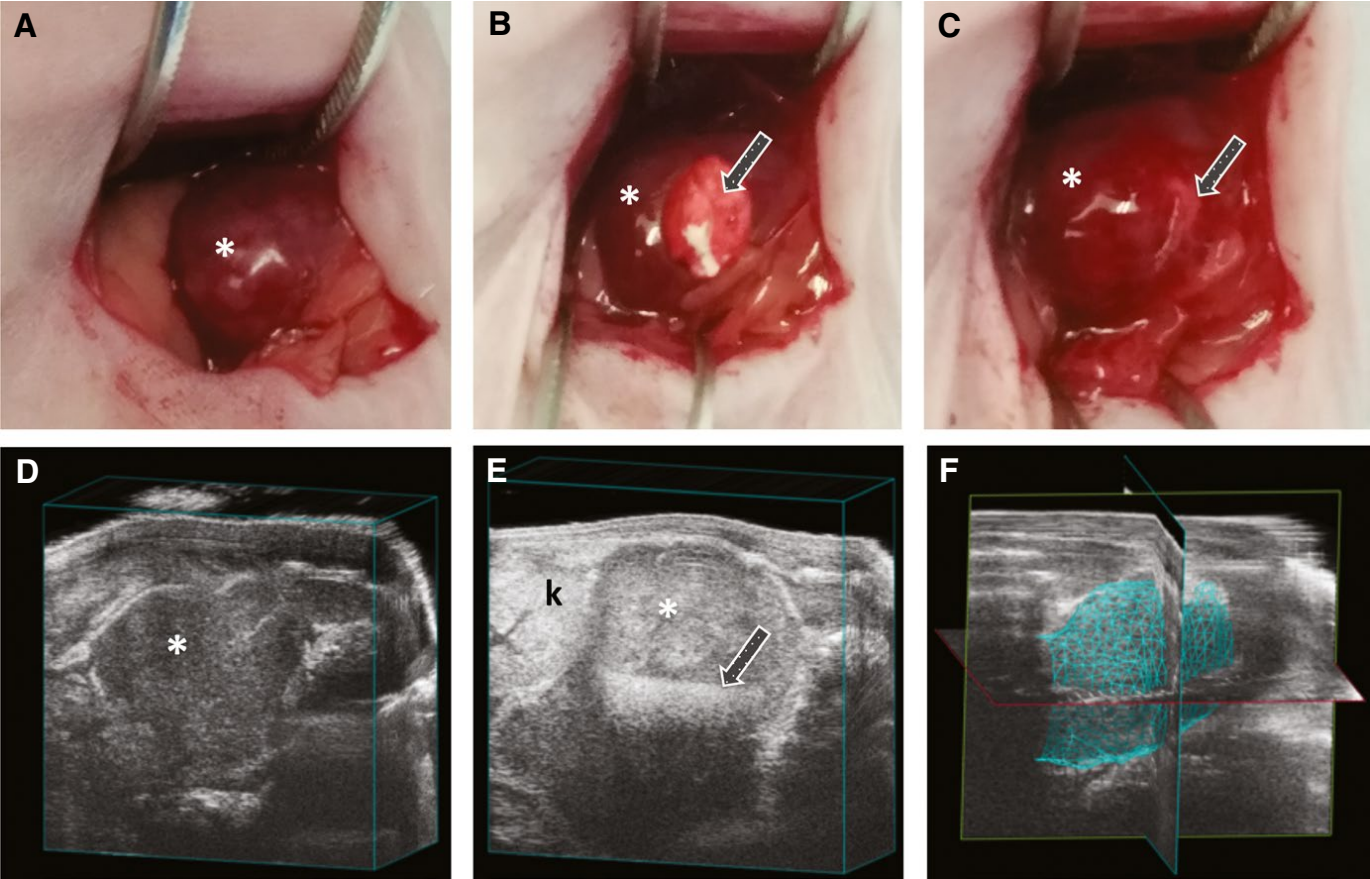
Implantation of silk foam into orthotopic neuroblastoma and ultrasound imaging. Orthotopic neuroblastoma (A) just prior to implantation of silk foam, (B) with silk foam halfway implanted and (C) completely implanted. Tumor growth/volume was followed with high‐frequency ultrasound (D) before and (E) after treatment with silk foam; (F) representative 3D image of tumor used to obtain volumetric measurements. Asterisk (*) indicates tumor location; arrow (→) denotes position of silk foam within the tumor; k indicates the left kidney
